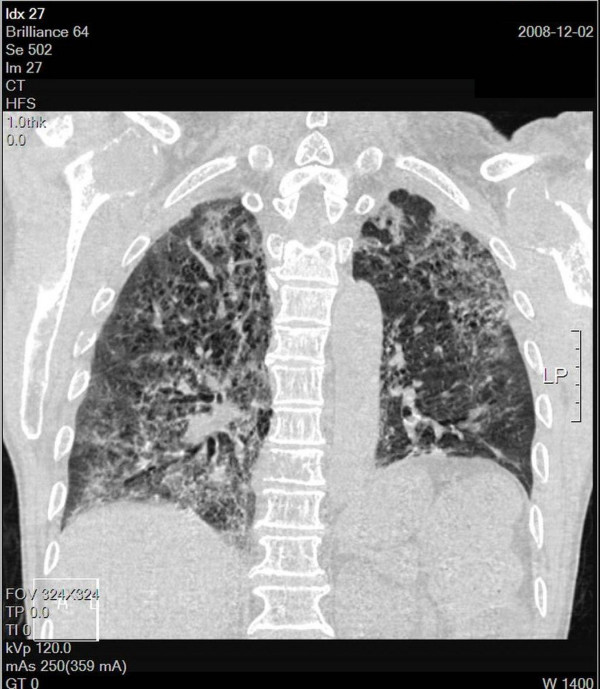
Chest computed tomography (CT) post intubation in the MICU shows interstitial pattern with traction bronchiectasis, opacities and a diffuse ground-glass pattern, bleb formation in marginal areas, airspace consolidation and fibrosis in the bilateral lung fields. The coronal views of chest CT.
